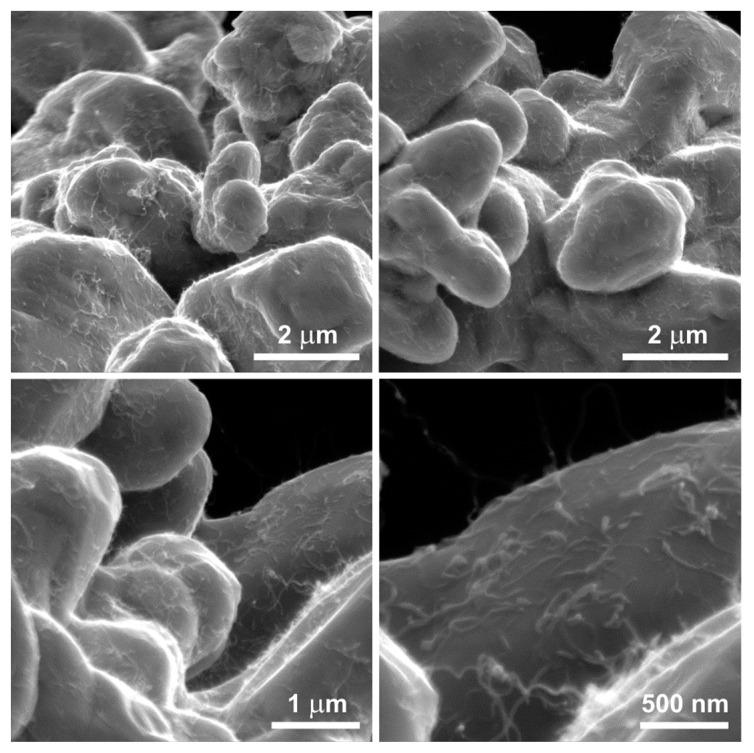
The structure of the 0.5 wt.% MWCNT/Cu powder containing chemically modified nanotubes, mixed in an ultrasonic chamber and a ceramic mortar grinder.
